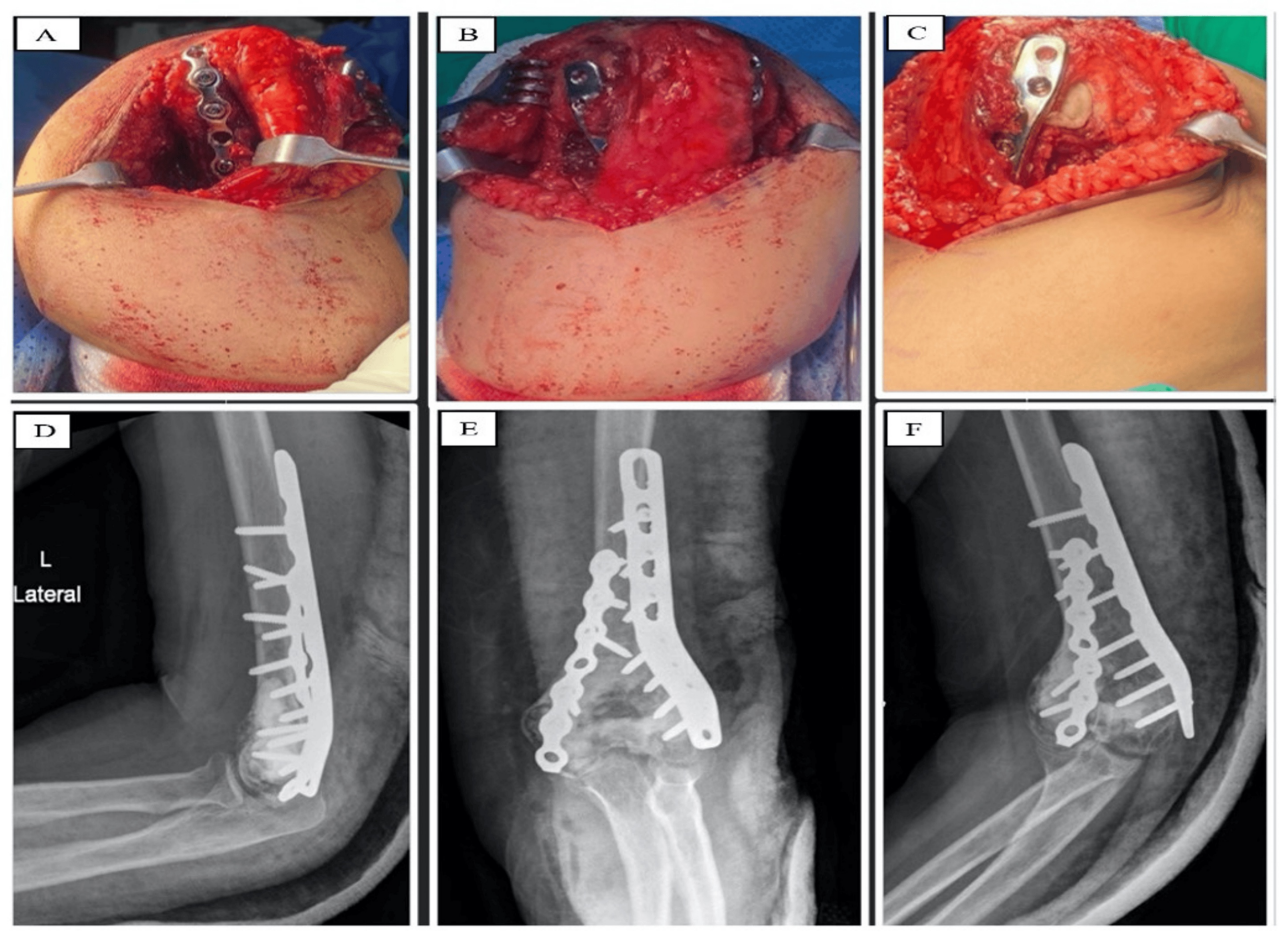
Surgical site with plates, cement application, and postoperative X-ray. (A) The surgical approach with the implant in place from the medial side. (B) The surgical approach with the implant in place from the posterior side. (C) The surgical approach with the implant and cement in place from the lateral side. (D) An X-ray lateral view of the elbow postoperatively. (E) An X-ray AP view of the elbow postoperatively. (F) An X-ray oblique view of the elbow postoperatively. AP: anterior-posterior
